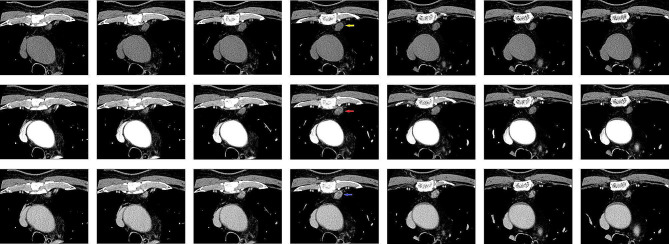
1.6 × 1 × 0.9 cm mass (Red /yellow/blue arrow) in the thymus region with CT scan. The plain CT (First line pictures) value was 25 HU, and the enhanced CT values were 34 HU (Arterial phase, Second line pictures) and 76 HU (Venous phase, Third line pictures)
